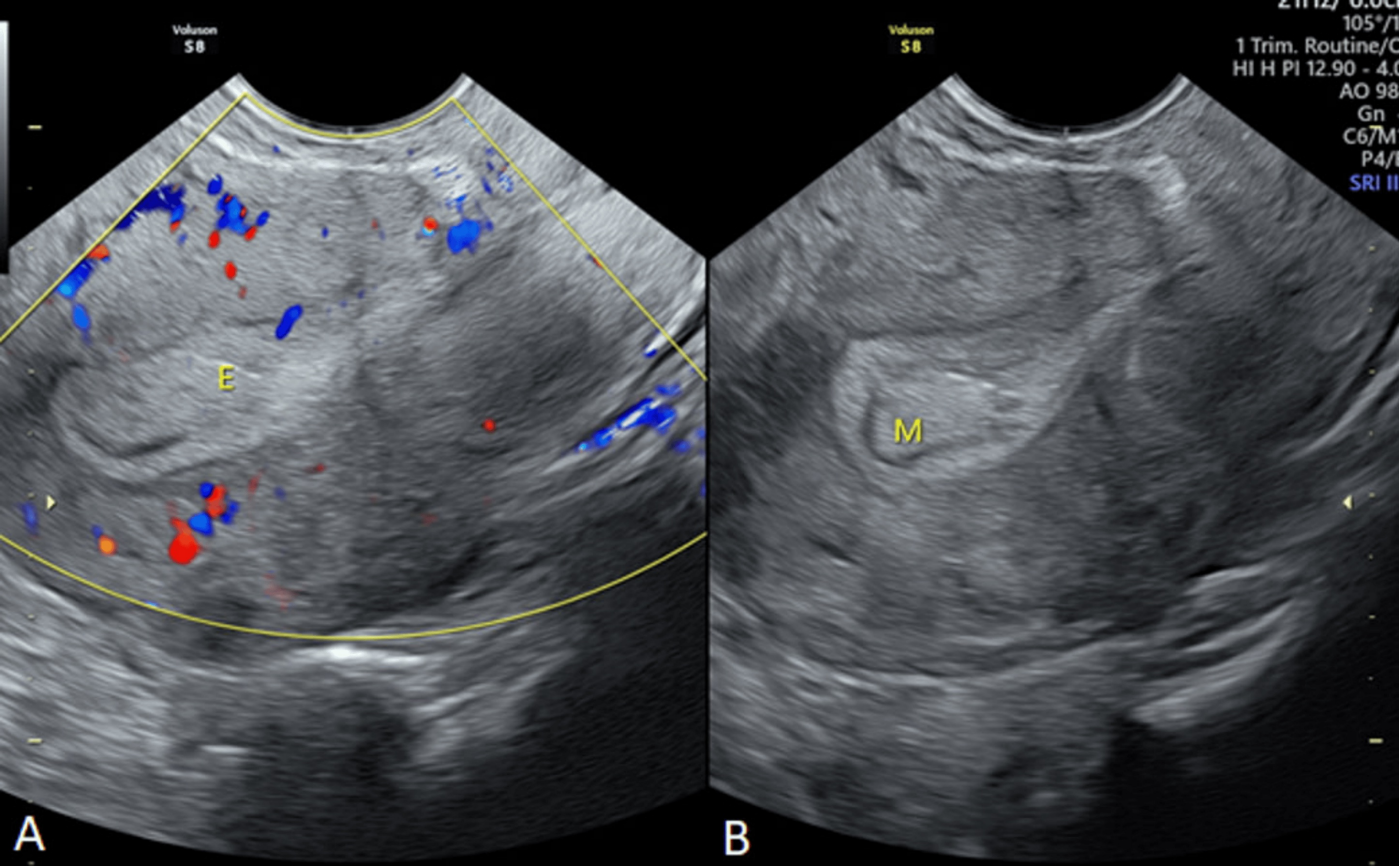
Transvaginal ultrasonography (TVS). (A) Color Doppler flow image of the endometrial lesion. (B) Grayscale image of the endometrial lesion in a female, 27 years old, with abnormal menstruation for more than two months. The postoperative pathology was an endometrial polyp, and the total score of the International Endometrial Tumor Analysis (IETA) ultrasound characteristics simple scoring method was 3 points (endometrial thickness 12 mm: 0 points; heterogeneous without cysts: 1 point; regular endometrial–myometrial junction: 0 points; having “Bright edge” sign: 0 points; color score: sparse vascularity: 2 points; single vessel (without branching): 0 points).
